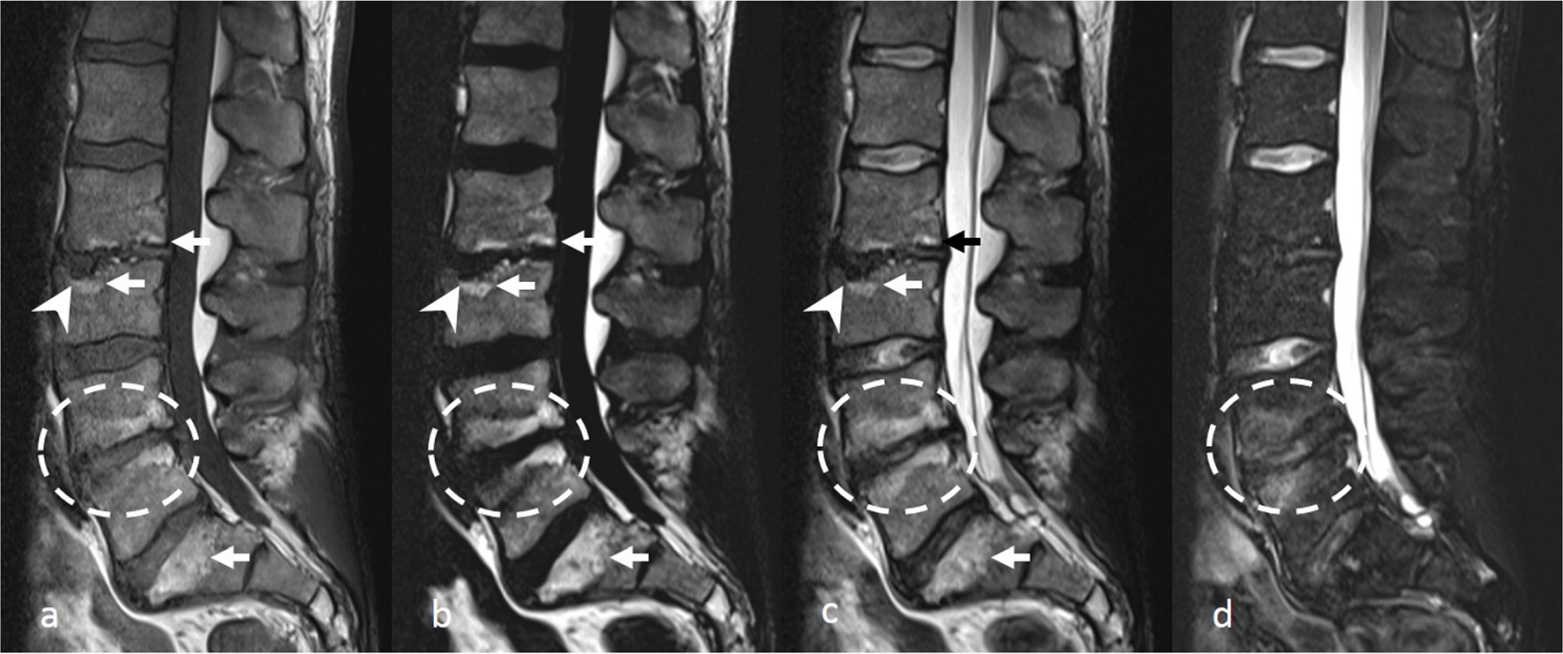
Lumbar spine MRI in a 38-year-old male including T1-weighted (a), fat-only image (b), in-phase image (c), and water-only image (d) from T2-weighted Dixon sequence. Fatty Modic type 2 changes are visible along the vertebral endplates adjacent to L2-L3 disc and superior endplate of S1 on a–c (arrows). Mixed Modic changes, combining predominantly inflammatory changes anteriorly (as seen on d), and fatty changes posteriorly are seen along the vertebral endplates adjacent to L4-L5 disc (dashed circles). Note the high contrast between fatty changes and surrounding tissues on b. Schmorl’s node is seen at superior endplate of L3 vertebral body (arrowhead), well depicted on a–c
